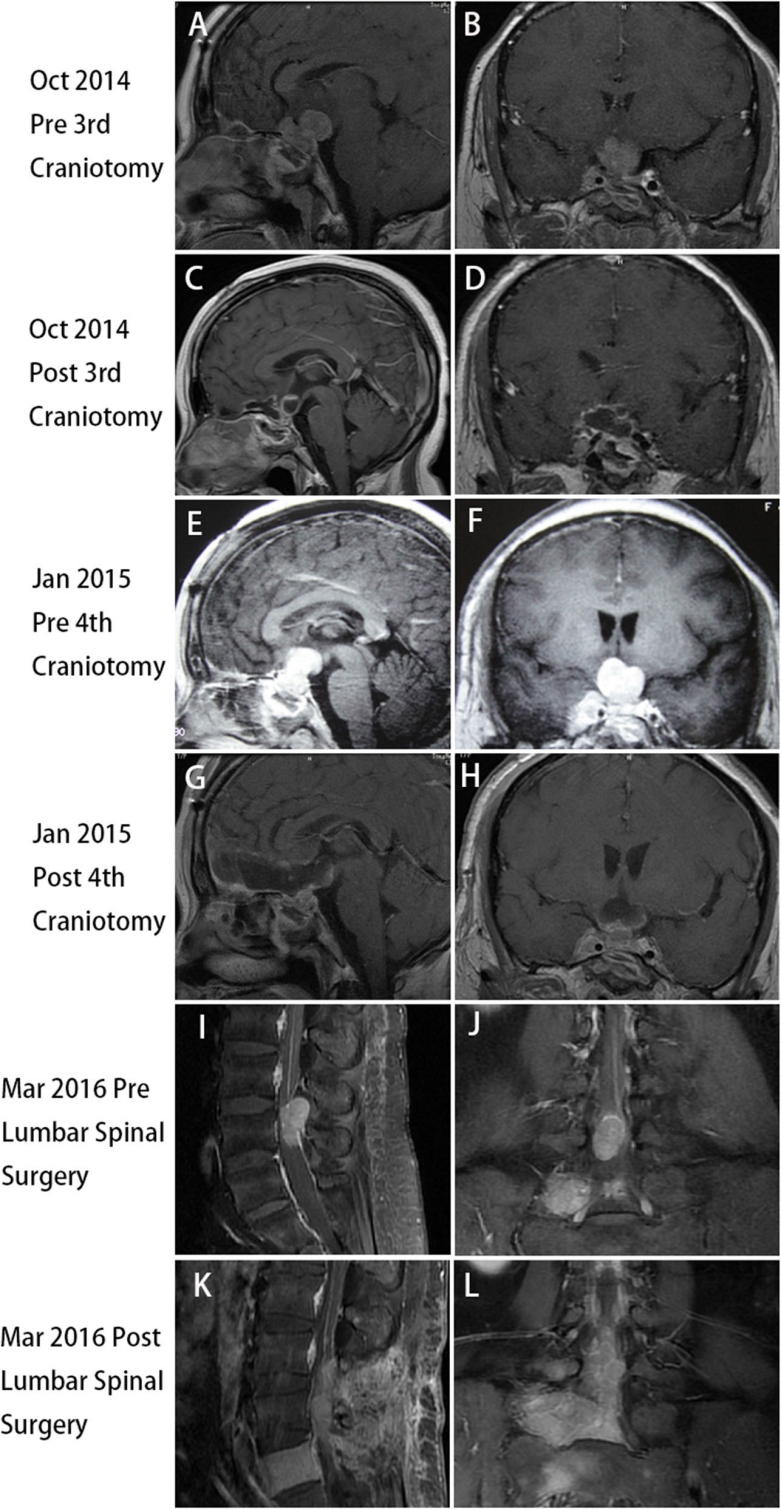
(A) and (B) Before the third frontal craniotomy, the MRI in October 2014 demonstrated a rapid enlargement of the tumor with suprasellar extension and encasing the right ICA. (C) and (D) After the third craniotomy, the tumor was subtotally removed. (E) and (F) Before the fourth frontal craniotomy, MRI in January 2015 reported that the tumor volume increased significantly and compressed the optic chiasm. (G) and (H) After the fourth craniotomy, the tumor was subtotally resected. (I) and (J) MRI of the lumbar spine indicated an intradural extramedullary mass at the level of the fourth lumbar vertebra. (K) and (L) Postoperative MRI indicated that the intradural extramedullary lesions were completely resected
